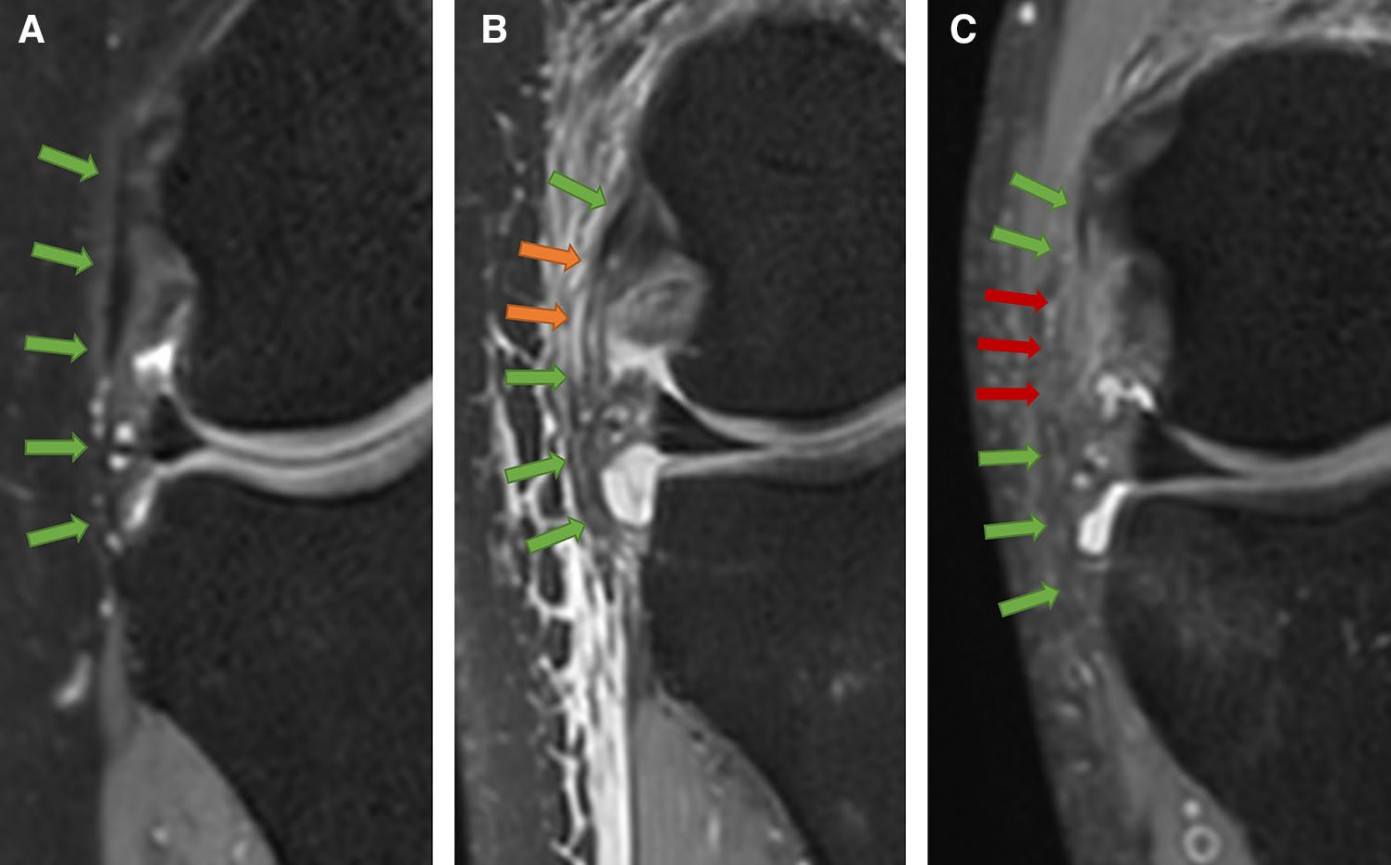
An example of each category proposed by Muramatsu et al. based on MPR MRI images. The ALL was rated as A “intact” if a continuous, clearly defined low-signal band was visible, or as B “strained” if “warping”, “thinning”, or “iso-signal changes” was visible or as C “torn” if no clear continuity was visible. The intact part of the ALL is indicated by the green arrows, the strained part by the orange arrows and the torn part by red arrows
